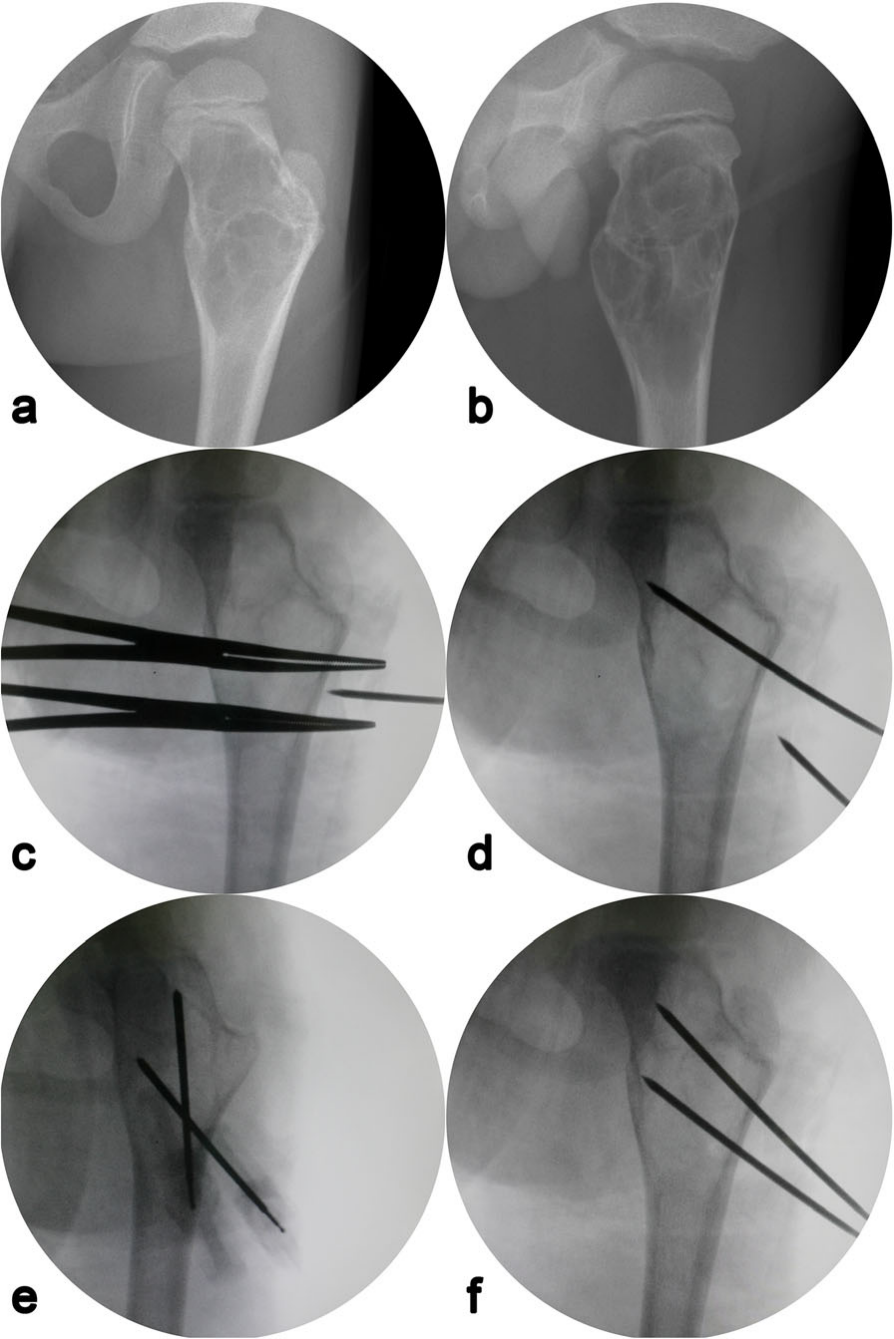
Radiographs of a 6-year-old boy during the operation. a, b Anteroposterior and lateral radiology manifestation of the cyst before puncture. c–f Under fluoroscopic guidance, two puncture needles were used to puncture 1/3 to 2/3 of the length of the cyst successively
